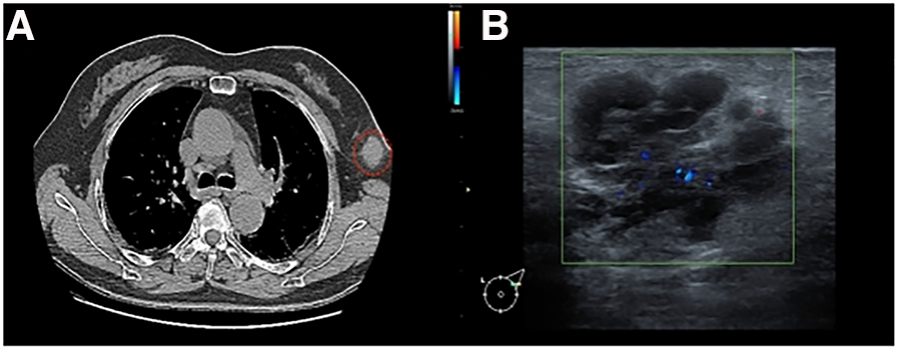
(A) Chest CT image: the red circle indicates a mass of the left accessory milk, which is not clearly connected to the breast tissue. (B) Mammary ultrasound: A glandular echo with a thickness of approximately 0.8 cm can be seen in the left axilla, and a hypoechoic nodule measuring approximately 3.0 cm × 2.1 cm can be seen on the inside. The boundary is clear and irregular in shape, with early lobulation, and the internal echo is uneven and separated.
